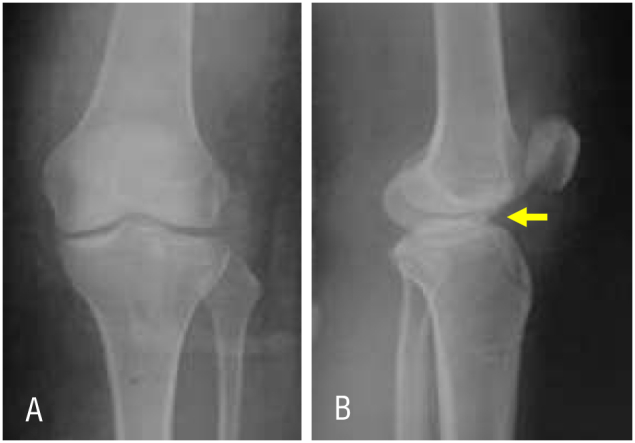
(A and B). Postoperative radiologic X-ray of the left knee shows anatomical reduction of the fragment (yellow arrow). (For interpretation of the references to colour in this figure legend, the reader is referred to the web version of this article.)
